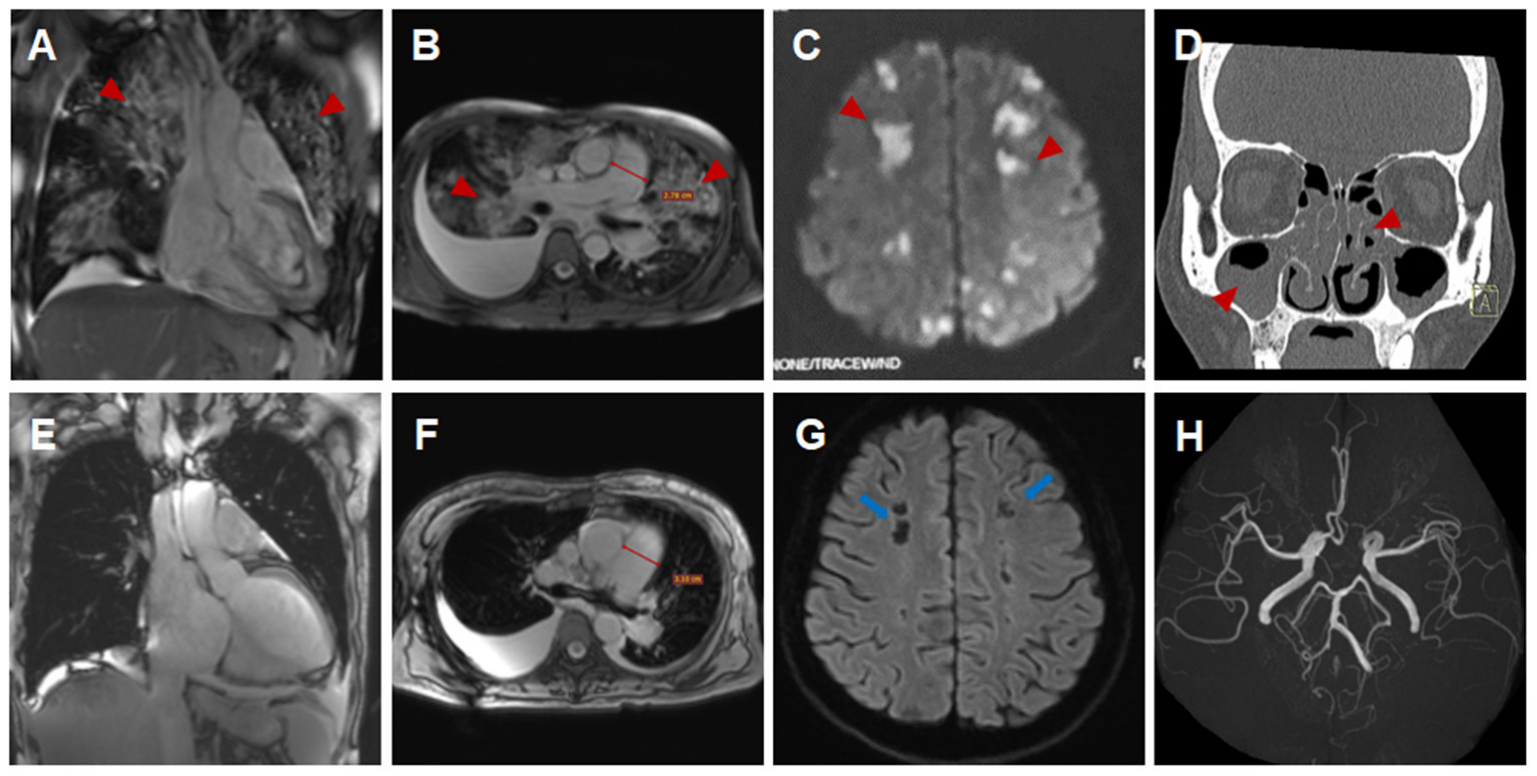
Imaging findings of eosinophilic granulomatosis with polyangiitis (A–D,H) Imaging findings during the initial hospitalization (A,D, coronal view; B,C,H, transverse view). Multiple patchy consolidations of both lungs (A,B, triangular arrows) on CMR images, multiple acute cerebral infarctions (C, triangular arrows) with no obvious cerebrovascular stenosis (H, triangular arrows) on brain MR images and sinusitis and nasal polyps (D, triangular arrows) on reconstructed CT images. (E–G) Imaging findings during the subsequent hospitalization (E, coronal view; F,G, transverse view). Significantly absorbed multiple lesions of both lungs (E,F), the pulmonary hypertension with increased diameters of pulmonary trunk from 28 mm (B) to 32 mm (F) and multiple encephalomalacia (G, long arrows).
